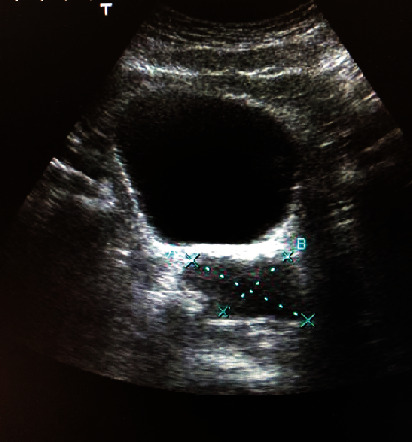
Abdominal ultrasound showing a cystic lesion close to the bladder.
